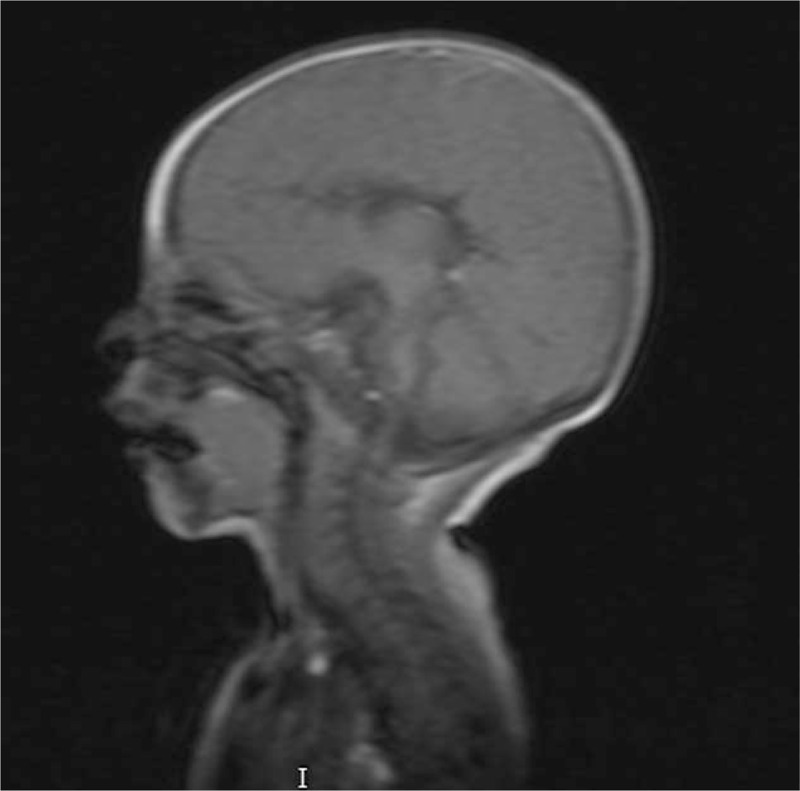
Brain Magnetic resonance imaging showing corpus callosum agenesis.
